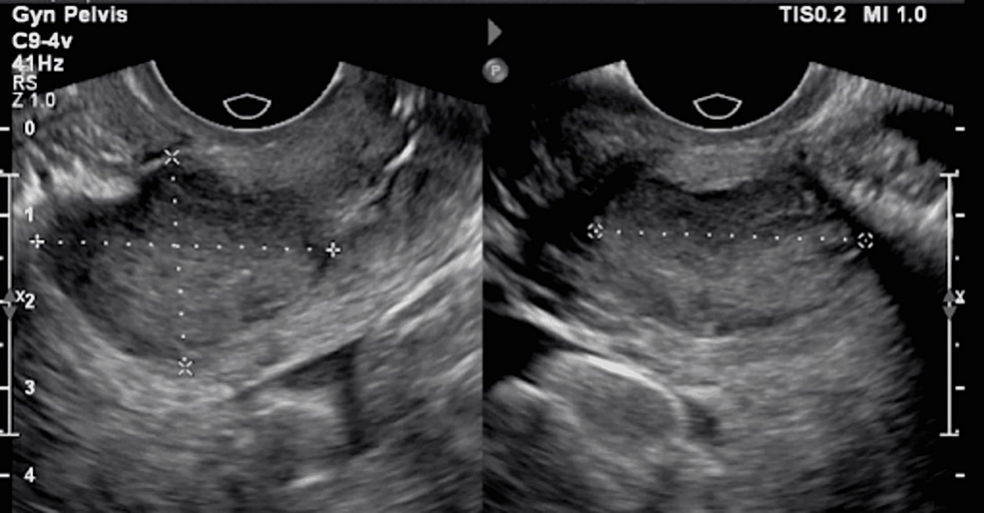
TVUS showing an irregular solid mass in the lower uterine segment measuring 3.5 × 2.45 × 3 cm. TVUS: transvaginal ultrasound
